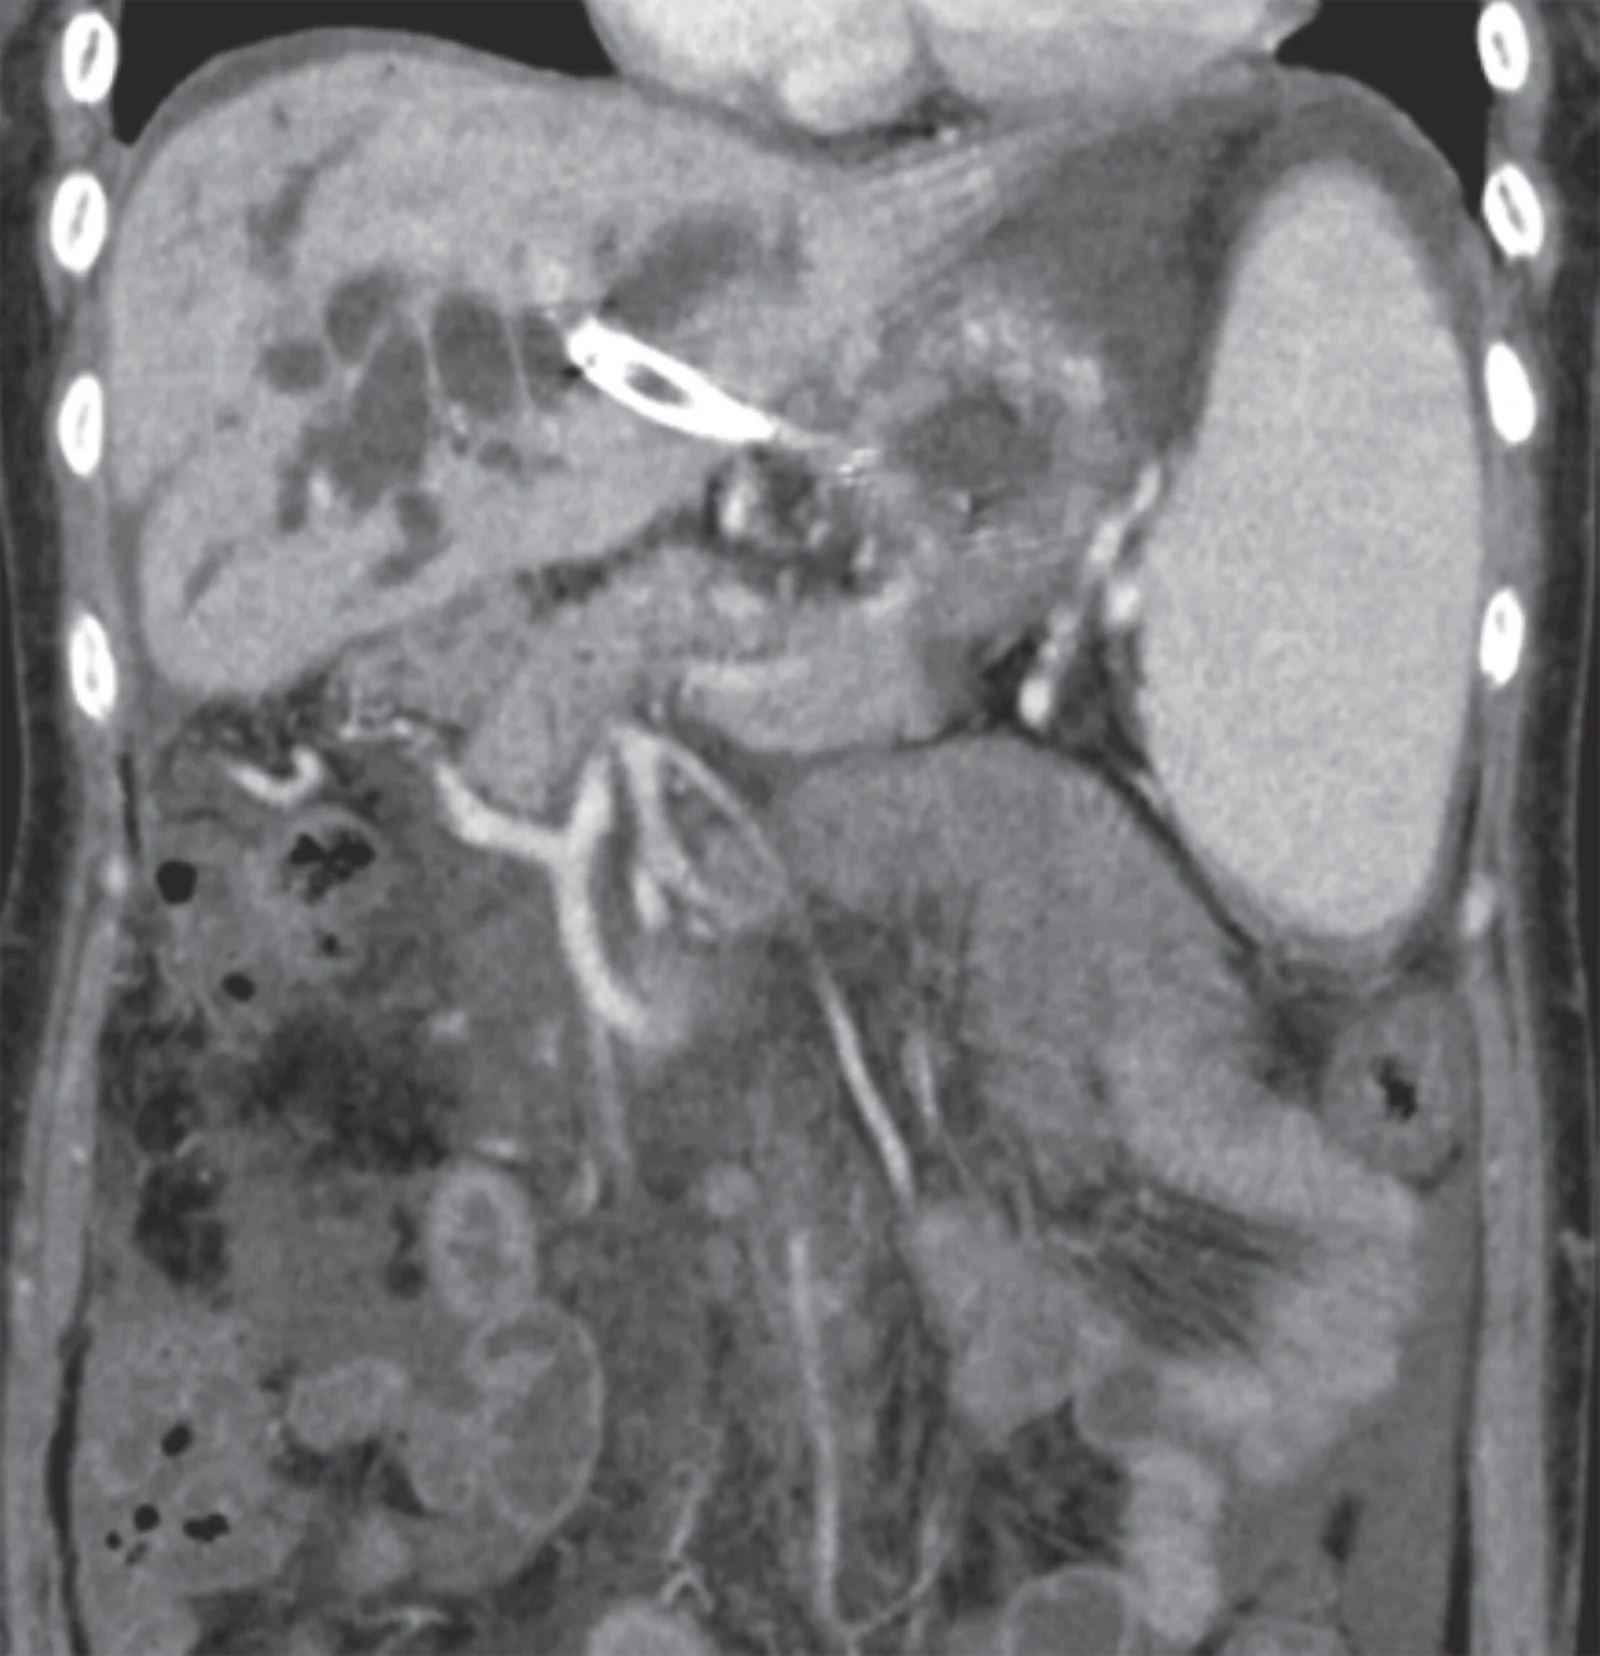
A coronal CT image showing intrahepatic bile duct dilat`ation
